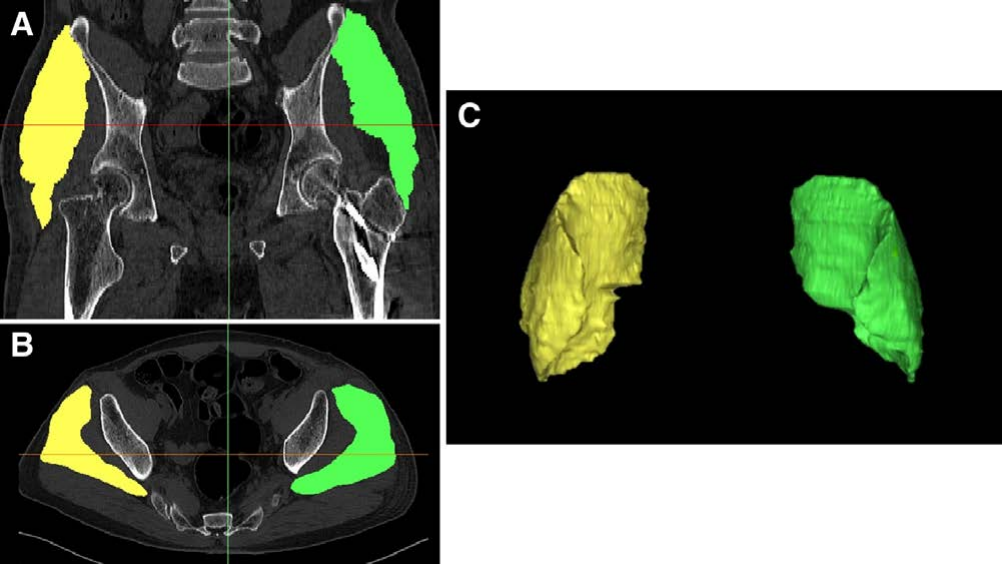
(A) Coronal computed tomography (CT) slice of both hips. Yellow, gluteus medius (GMED) of the uninjured side; green, GMED of the fractured side after surgery. (B) Axial CT slice from the same patient. (C) Three-dimensional reconstruction of bilateral GMED muscles.
